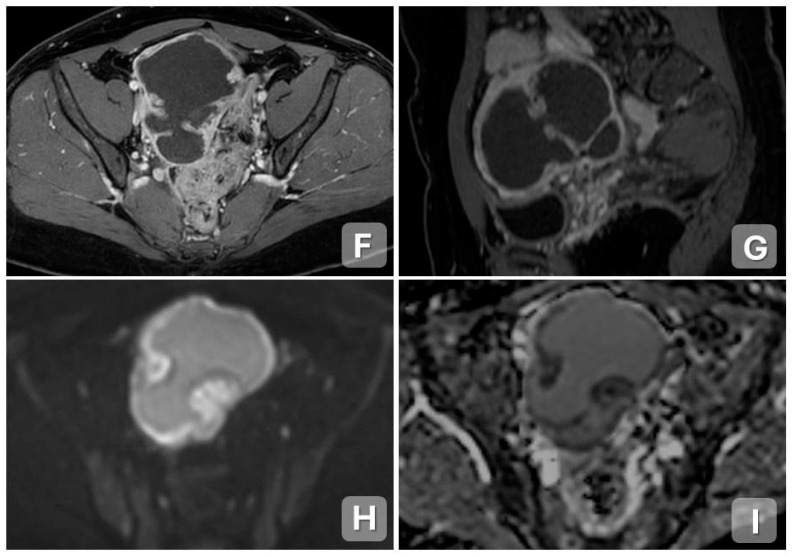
Abscessed tumor in a 35-year-old woman with fever and pelvic pain. (A–C) Sagittal, axial, and coronal T2-weighted MRI images demonstrate a complex right adnexal mass with thickened, irregular walls, internal vegetations, and heterogeneous content. (D–G) Axial and sagittal contrast-enhanced T1-weighted images show marked enhancement of the abscess wall and the solid components. (H,I) DWI and corresponding ADC map reveal pronounced diffusion restriction within the solid component, consistent with high cellularity.
